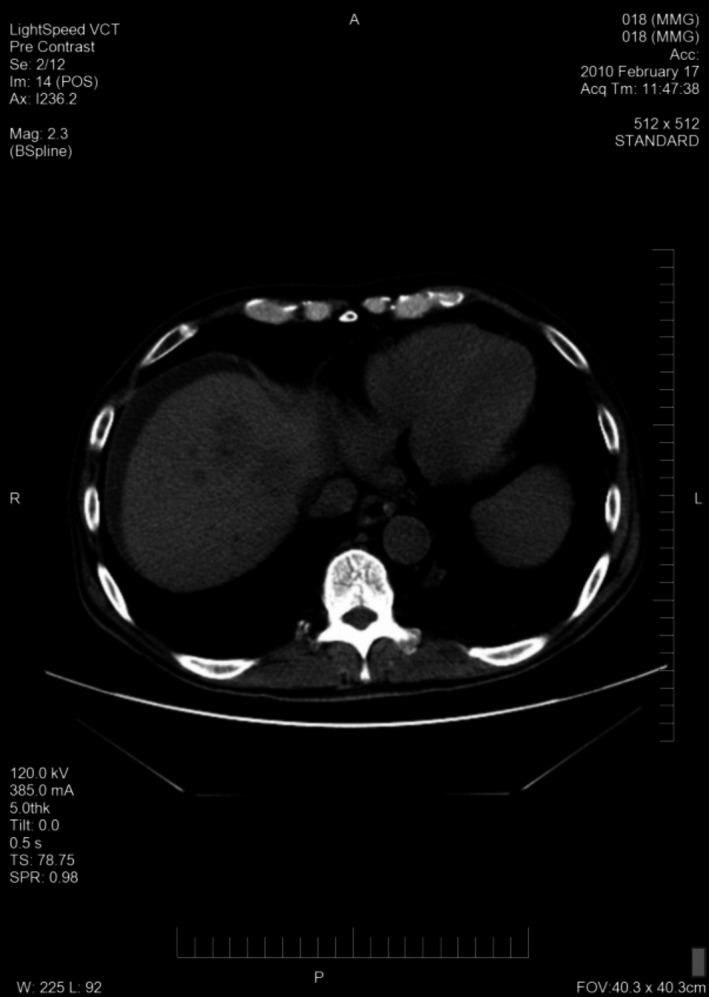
6‐month follow‐up CT image ( without contrast) of patient 1 with Hepatocellular Carcinoma (HCC) the maximal tumor diameter was 6.6 x 2.6 cm showing a good PR on trial.
